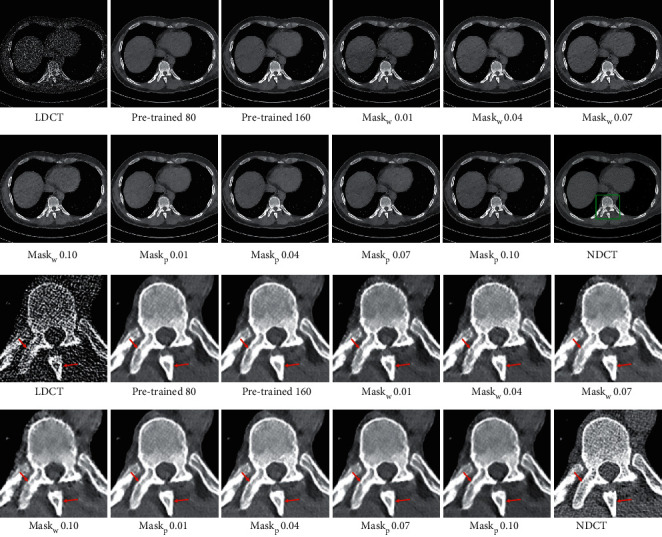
The denoised result for low-dose CT image with different threshold values and training strategies. The display window is [100, 300] Hu for better visualization. Pretrained 80 denotes the image generated by the model trained without local filtered mechanism for 80 epochs. Pretrained 160 denotes the image generated by the model trained without local filtered mechanism for 160 epochs. Both Mask_*w*_ and Mask_*p*_ denote the image generated by the model trained with local filtered mechanism. Mask_*w*_ denotes the image generated by the model trained without the pretrained model. Mask_*p*_ denotes the image generated by the model trained with the pretrained model.
